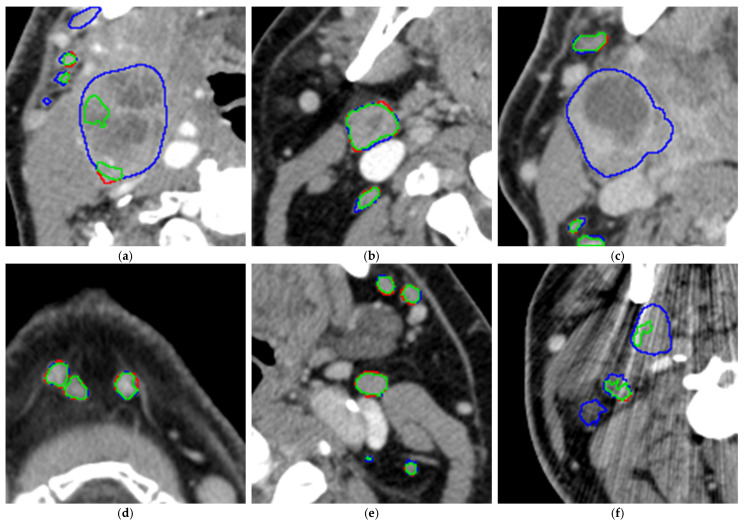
(a–f). Analysis of the segmentation overlays on 6 example CT slices (green: correct segmentations, blue: false-negative segmentations, thus manually segmented areas left out by the model, red: false-positive segmentations, thus areas segmented by the model but left out in the manual segmentations).
